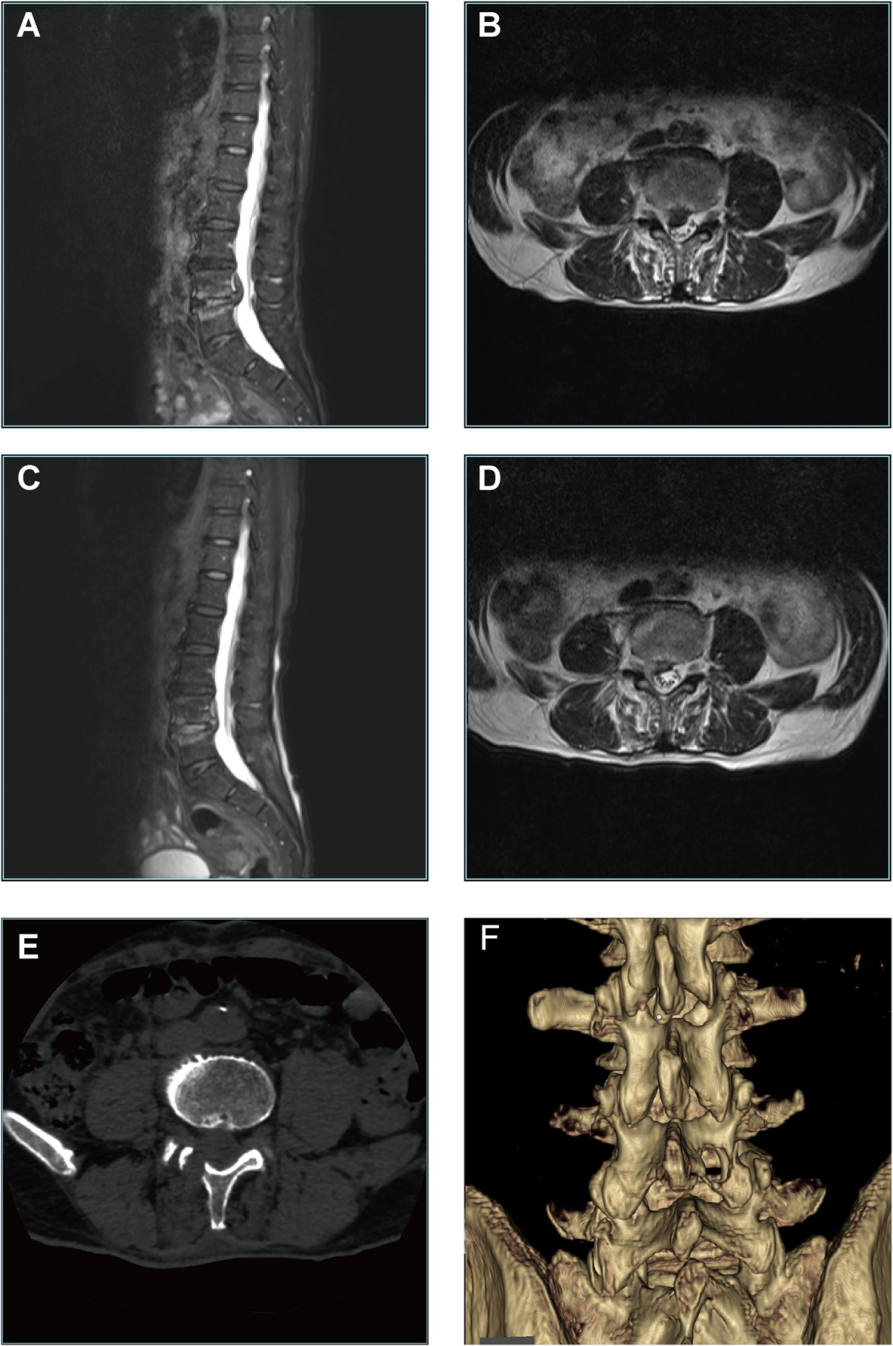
Case 1: a 74-year-old male patient was admitted to the hospital due to “pain and numbness in the right lower extremity for 19 h”. Lumbar MRI showed that the L4/5 intervertebral disc had migrated to zone 1 in the upper right part of the body (Fig. 6a, b). The patient underwent posterior PELD. During surgery, the herniated intervertebral disc was removed. MRI was repeated to confirm complete removal of the herniated disc in the spinal canal (Fig. 6c, d). Three-dimensional computed tomography (CT) reconstruction indicated that the bone tunnel was located at the lower edge of the lamina, which was consistent with the preoperative plan (Fig. 6e, f)
